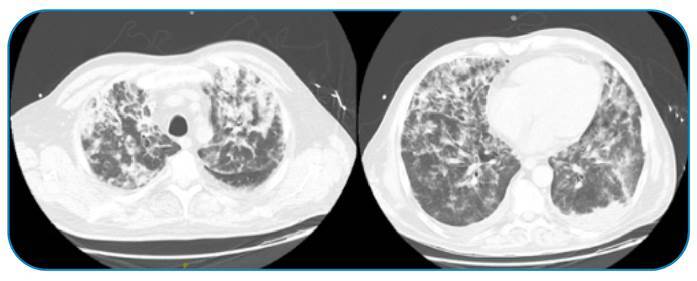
Computed tomography scan from hospital admission demonstrating patchy ground-glass opacities that are more evident in the lower and upper lobes, interspersed with zones of parenchymal consolidation.
